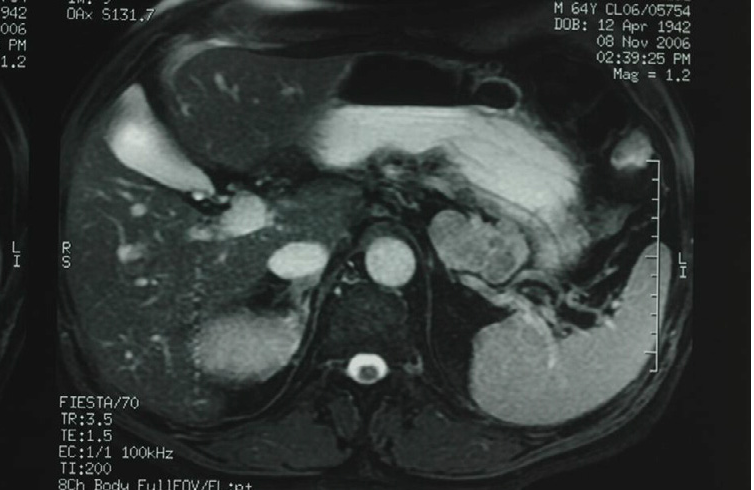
MRI of the cystic teratoma of the pancreatic tail. Enhanced magnetic resonance shows a cystic mass arising from the tail of the pancreas extending to the middle side of the stomach without defined margins.
